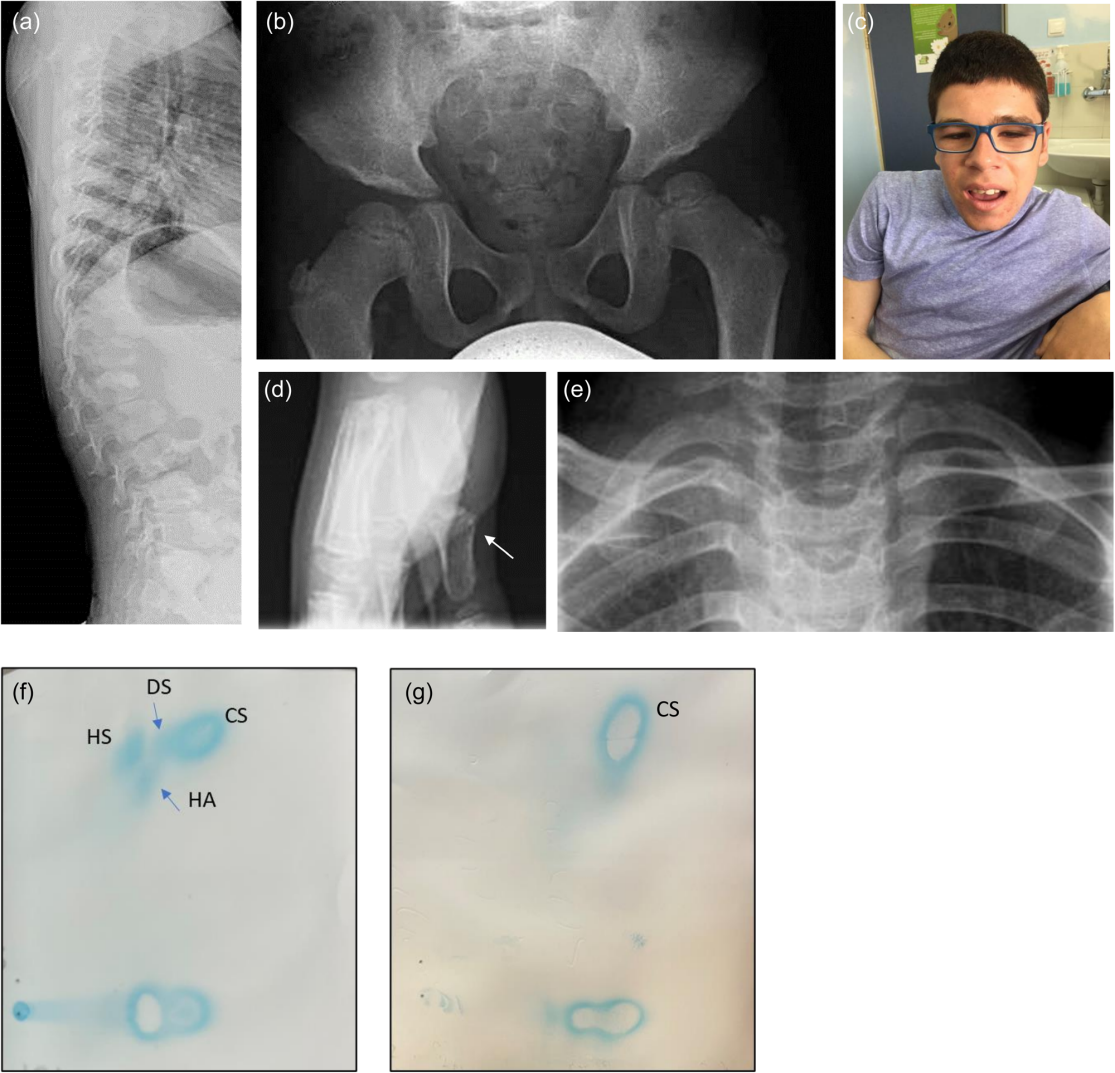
Skeletal complications and two‐dimensional electrophoresis of urinary GAGs in patients with a juvenile form of mucopolysaccharidosis plus disease. (a) Lateral spinal radiograph of patient P6 in middle childhood showing flattened and oval‐shaped vertebrae. (b) Radiograph of pelvic showing flattening acetabula obtained in early childhood. (c) Patient P6 in late adolescence showing coarse facial features, ptosis, short neck. (d) Radiograph of foot in early childhood: widening of metatarsal bones. (e) Broad and short claviculae on cervical spine radiograph of patient P6 in late childhood. (f) Patient P6 urinary GAG pattern showed increased heparan (HS), some dermatan (DS) sulphates, and hyaluronic acid (HA). (g) Healthy individual with normal pattern of GAG. GAG, glycosaminoglycan.
